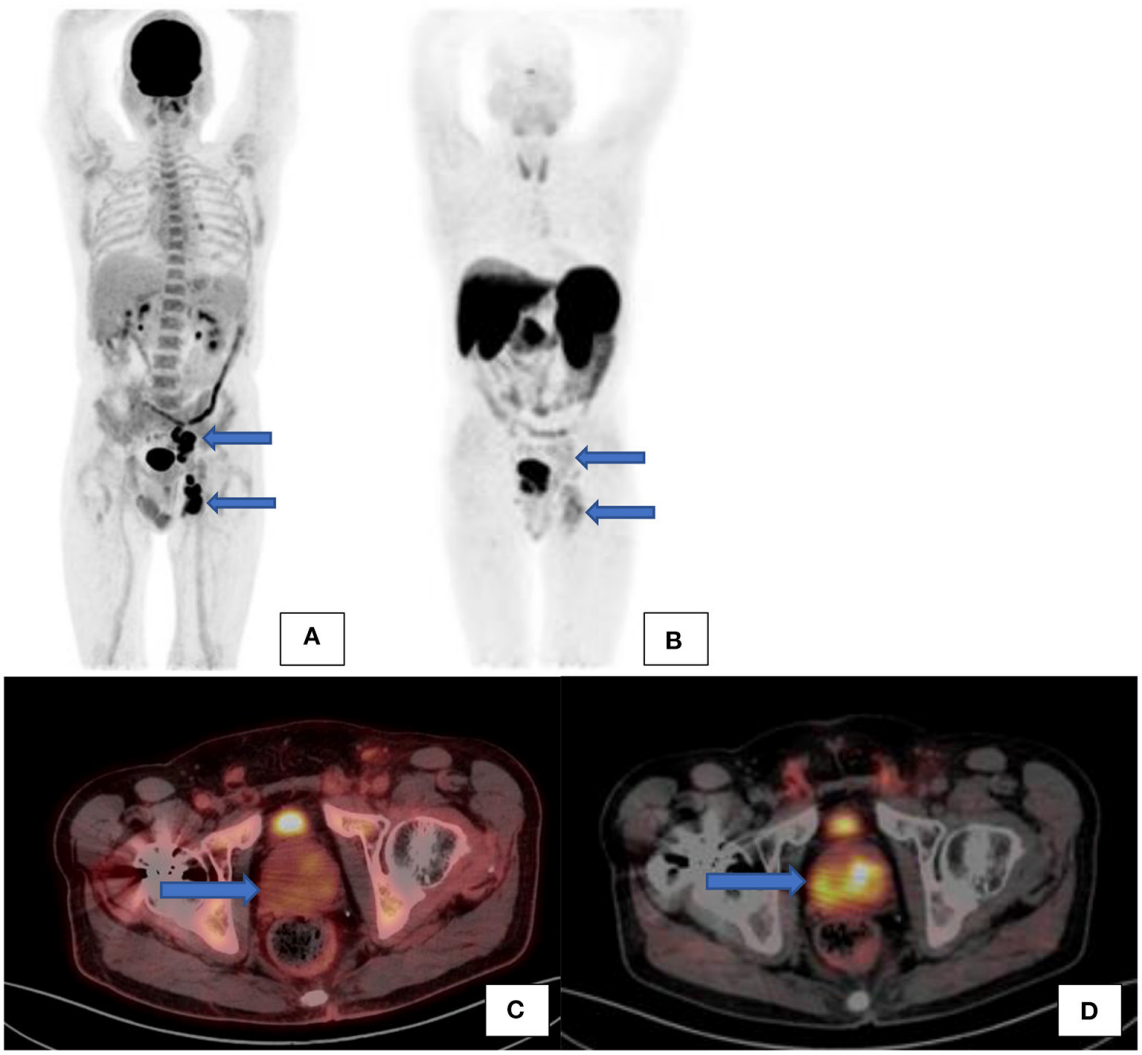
A patient with confirmed prostate carcinoma underwent a Ga68-PSMA scan (see the maximal intensity projection image (B) and transaxial fused PET/CT image (D) showing avid uptake in the prostate gland and low grade uptake in the left internal iliac and inguinal nodes. F18-FDG scan [(A)–maximal intensity projection images and (C)–transaxial fused PET/CT image] was also performed on the same patient showing low grade uptake in the prostate gland but avid uptake in the same left internal iliac and inguinal nodes.
